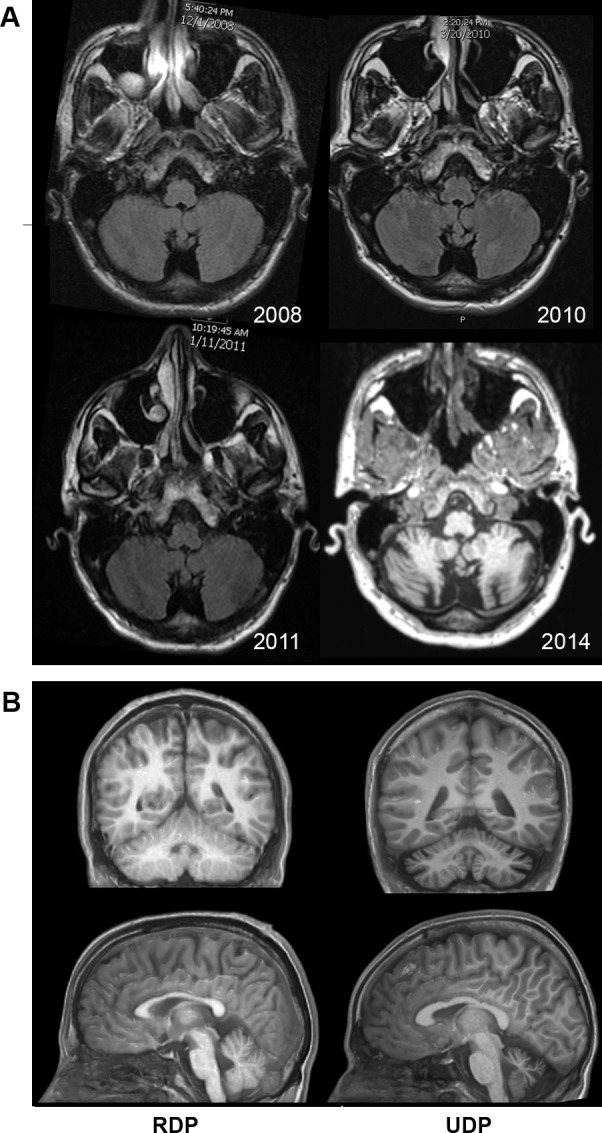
Sequential and comparative MRI. (A) Axial T1-weighted images from successive MRI scans acquired from 2008 (year of onset) to 2014 demonstrate the progressive development of cerebellar atrophy. (B) Coronal (top row) and sagittal (bottom row) T1-weighted images from MRI demonstrate relatively normal cerebellar structure and volume of a representative RDP patient (left images) in contrast to marked cerebellar atrophy of the patient in 2014 (right images).
